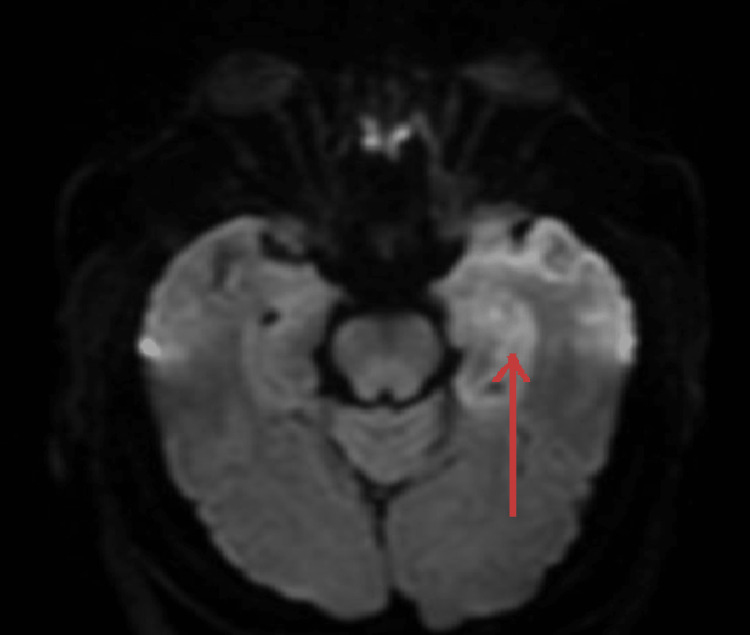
MRI scan, diffusion-weighted imaging phase MRI of the patient showing restricted diffusion involving the left temporal lobe and left insular cortex.
